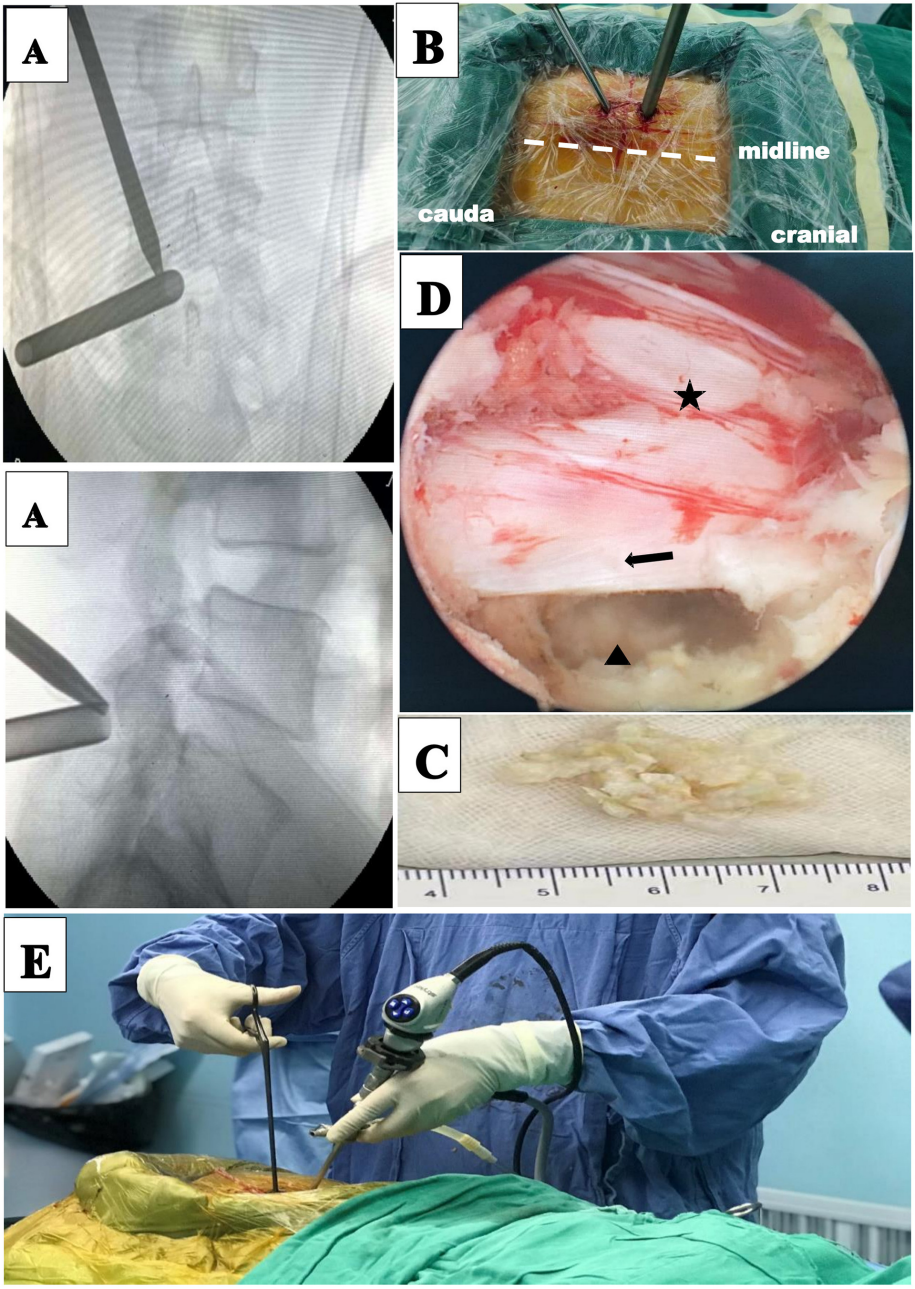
Unilateral biportal endoscopic discectomy (UBED) for a 69-year-old female patient with L4–L5 disc herniation. (A) Intraoperative anteroposterior x-ray shows the locations of the two cannulas. (B) Portal placements for the UBED. (C) Disc pulposus. (D) Intraoperative images: (star) dural sac, (arrow) nerve root, (triangle) disc. (E) Intraoperative overview of UBED.
